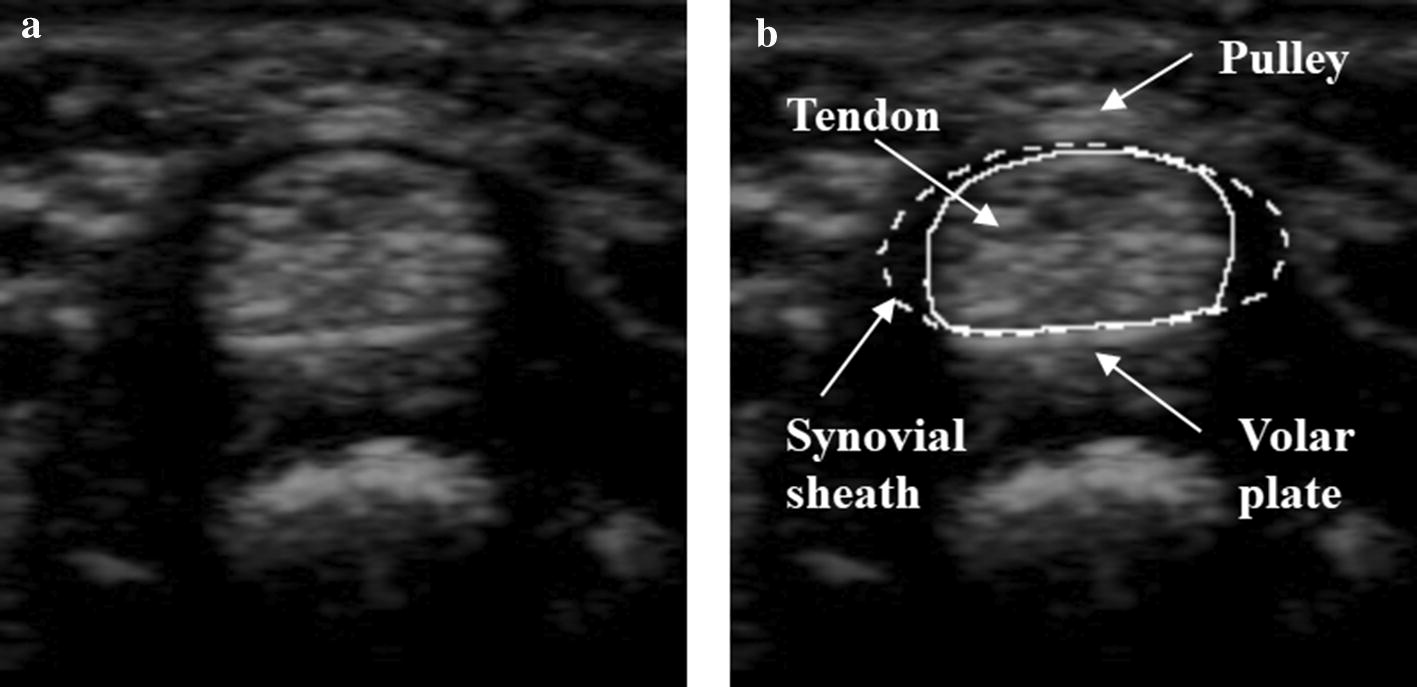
Ultrasound image of the tendon and synovial sheath of a finger. a Original image acquired around A1 pulley. b Tendon (solid line), synovial sheath (dotted line) area, and surrounding tissues
